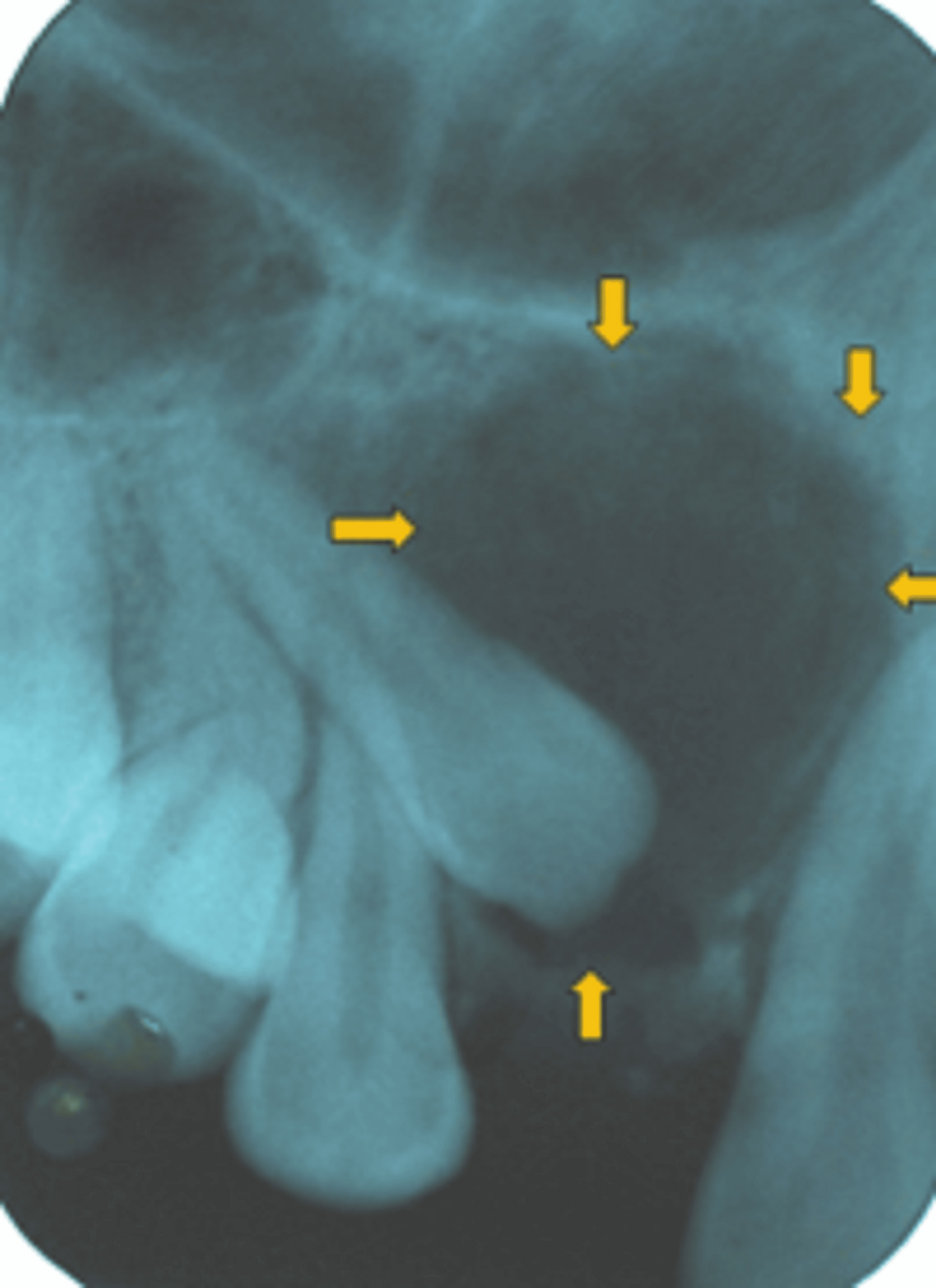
IOPA revealing pericoronal radiolucency associated with unerupted right maxillary lateral incisor IOPA: intraoral periapical radiograph
